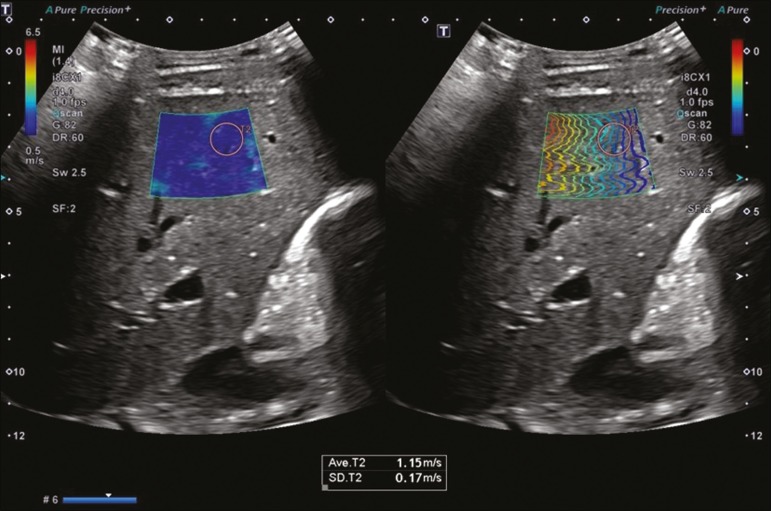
Ultrasound examination of a normal liver. A 2D-SWE study showing normal elasticity of the liver parenchyma (1.15 m/s), consistent with a METAVIR stage of F0. The elastography map is homogeneous and predominantly blue, a color range that represents less stiffness, and the propagation mode (on the right) indicates normality.
